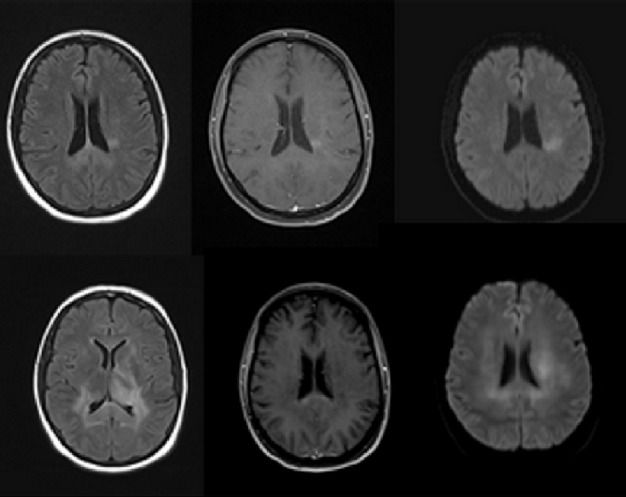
Comparison of initial and follow-up MR images Images from the initial *(top row) *and subsequent MRI *(bottom row) *demonstrating an additional site of lymphomatous involvement. Abnormal flair intensity *(1st column)* and abnormal patchy enhancement is seen on post-contrast images in the periventricular white matter and corpus callosum *(second column) *with corresponding DWI restriction *(third column).*
